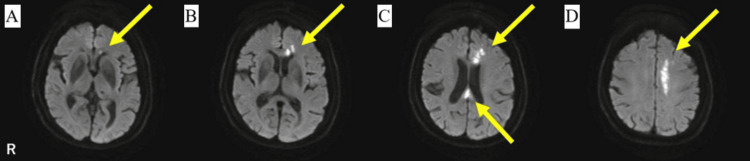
Diffusion-weighted MR images obtained 7 days after symptom onset (A-D) High-signal intensity regions are visible in the splenium, body, and genu of the corpus callosum (yellow arrows). MR: magnetic resonance
